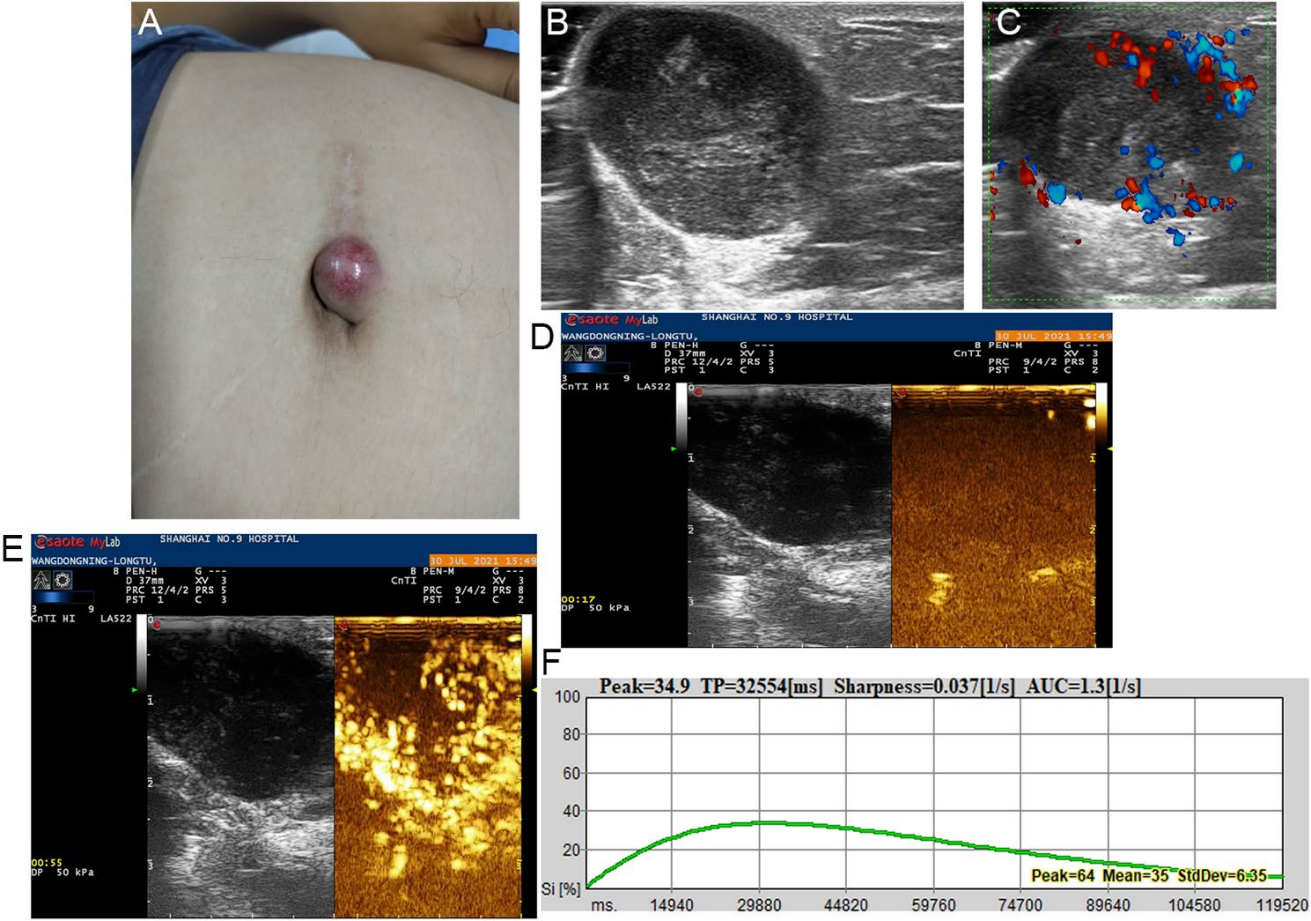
A Recurrent DFSP with red nodule in the abdominal wall of a 36-year-old man. B Transverse ultrasonogram revealing a well-defined, hypoechoic, heterogeneous, and subcutaneous lesion. C Color Doppler ultrasonogram revealing rich vascularization with vascular densities reaching 4.5/cm^2^. D Contrast-enhanced ultrasound revealed the trend that contrast agent enters the lesion from the periphery to the center. E Contrast-enhanced ultrasound revealed heterogeneous hyper-enhancement at peak (the 32th second), with filling defect in the center. F Time intensity curve revealed peak of 34.9 and sharpness of 0.037
